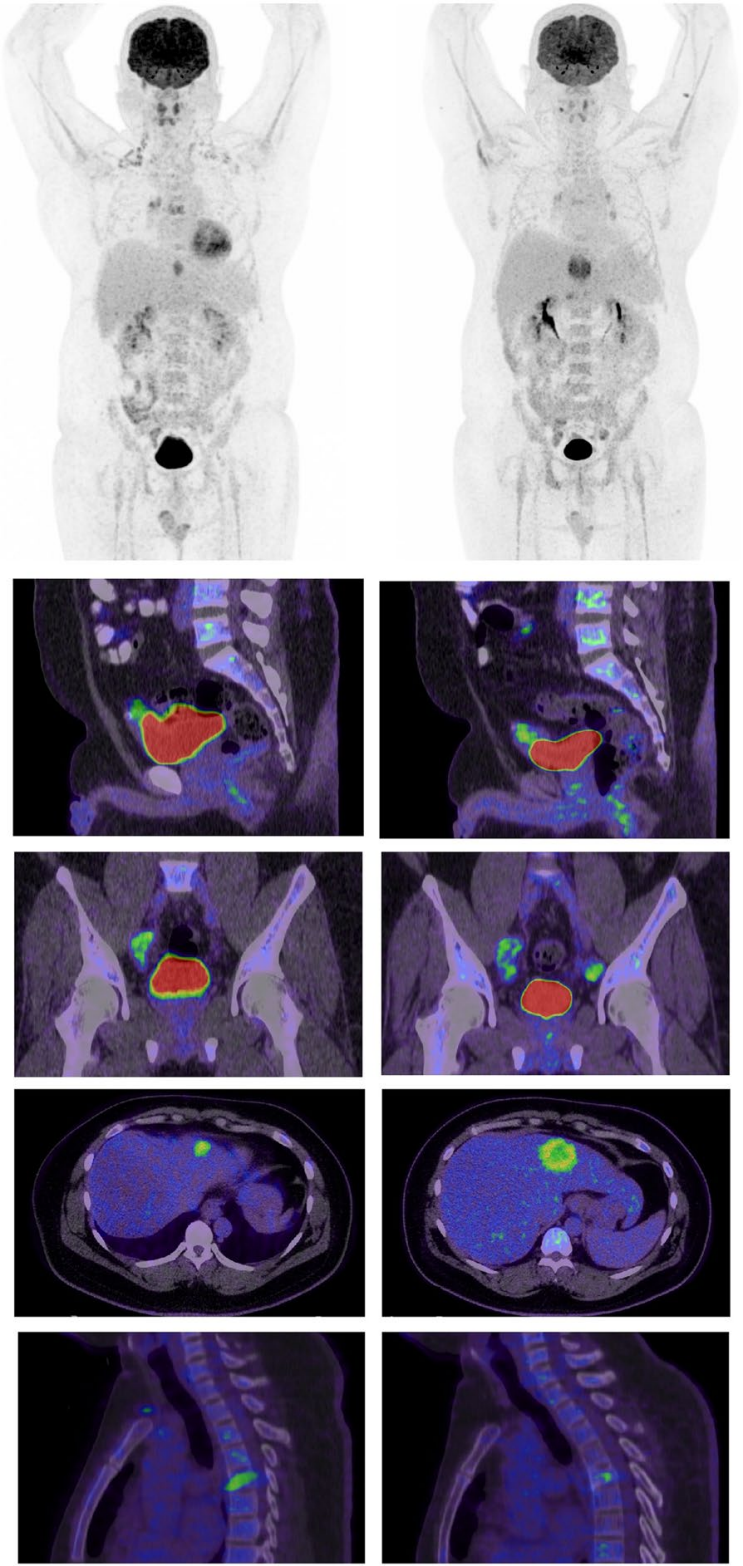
Staging (left column) and restaging (right column) FDG PET/CT scan maximum intensity projection (MIP—A-B) and fused sagittal (C-D and I-J), coronal (E–F) and transaxial (G-H) images. The primary tumor in the ventral aspect of the urinary bladder (red arrowhead) was seen even with the presence of the excreted FDG in the bladder. Bilateral parailiac lymph nodes showed progression during treatment (blue arrowheads). Liver metastasis in the left lobe has also increased in size (green arrowhead). Irradiated D5 vertebral body lesion partially responded (yellow arrowhead). Physiologic brown fat activity was noted on the staging scan (brown arrowheads)
